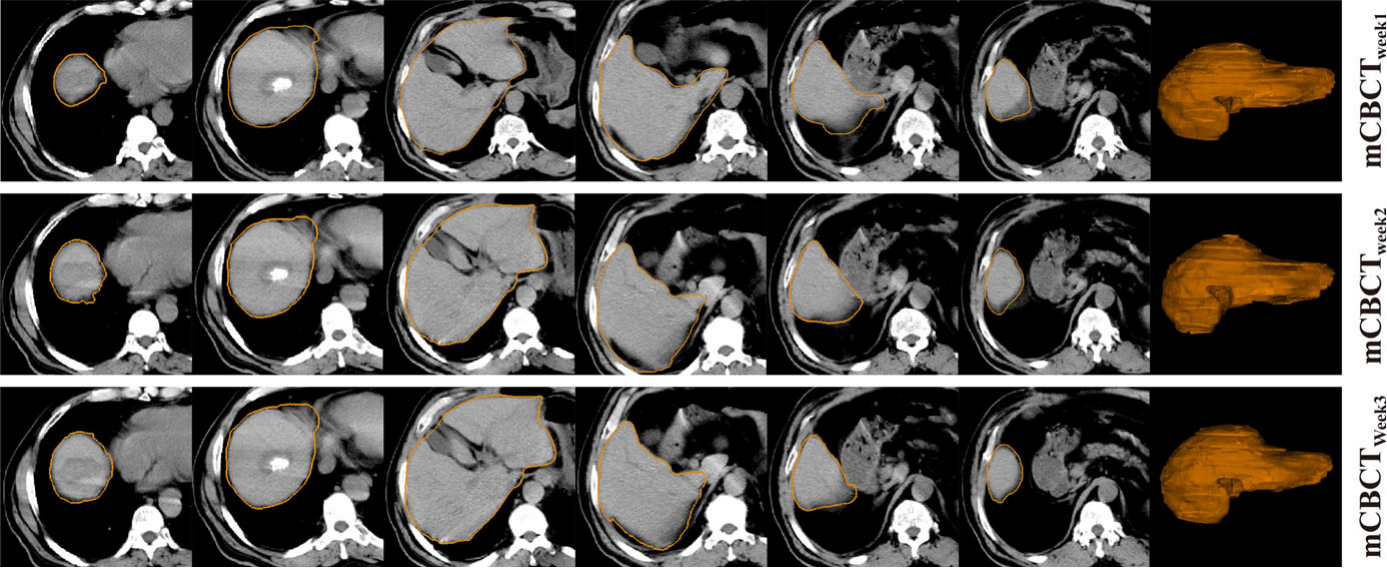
Example of slice and three‐dimension region for a sample segmented using deformable registration propagated methods for mCBCTweek1, mCBCTweek2, and mCBCTweek3. The subset of slices was arbitrarily selected by increasing the slice numbers to approximately cover the entire liver volume.
